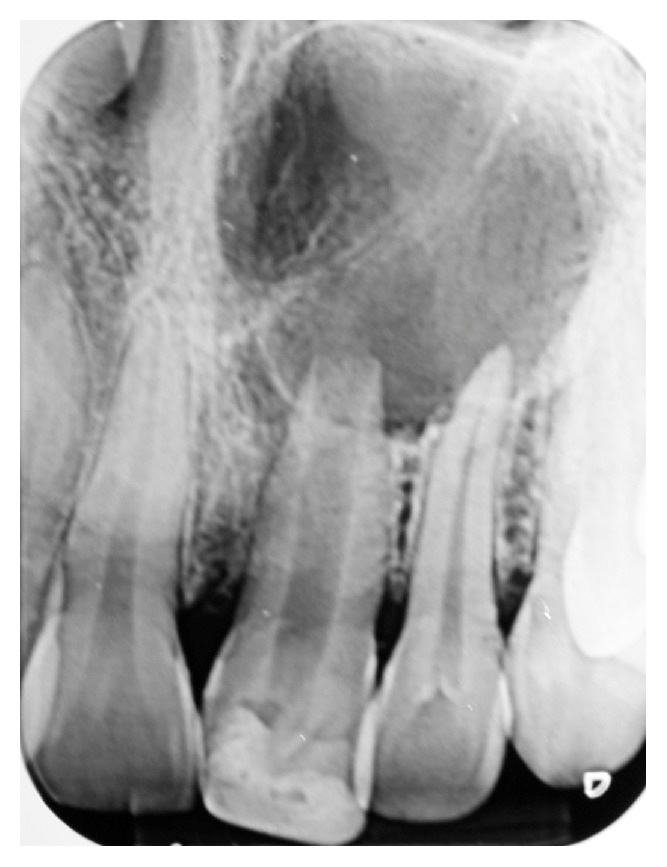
Initial radiograph of the maxillary right central incisor.
